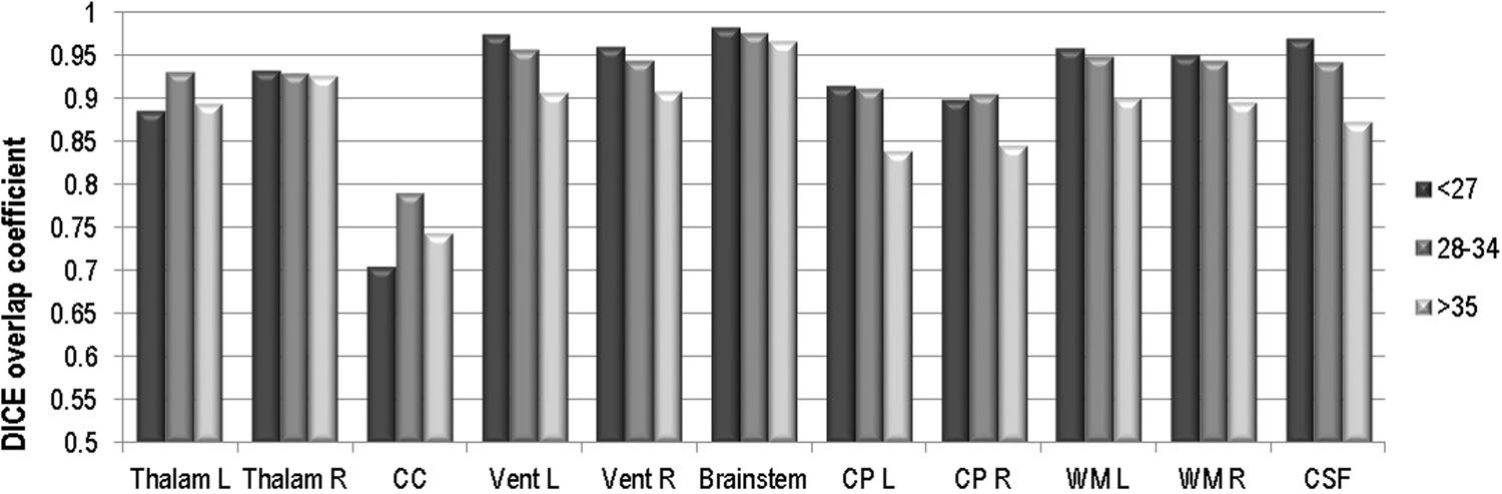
DSC metrics computed for brain tissue and structures for leave-one-out automatic multi-atlas segmentation evaluation applied to seven subjects with reference manual segmentations (the test set), averaged in three GA ranges: less than 27 weeks, between 28 and 34 weeks, and more than 35 weeks. Overall, the results indicated that relatively accurate automatic segmentation of the main brain structures and tissue were achieved by using the developed spatiotemporal fetal brain MRI atlas, symmetric diffeomorphic deformable registration for label propagation^60^, and probabilistic STAPLE^65^ for label fusion. Labels are: Thalam L: left thalamus, Thalam R: right thalamus, CC: corpus callosum, Vent L: left lateral ventricle, Vent R: right lateral ventricle, Brainstem, CP L: left cortical plate, CP R: right cortical plate, WM L: left developing white matter, WM R: right developing white matter, and CSF: cerebrospinal fluid.
